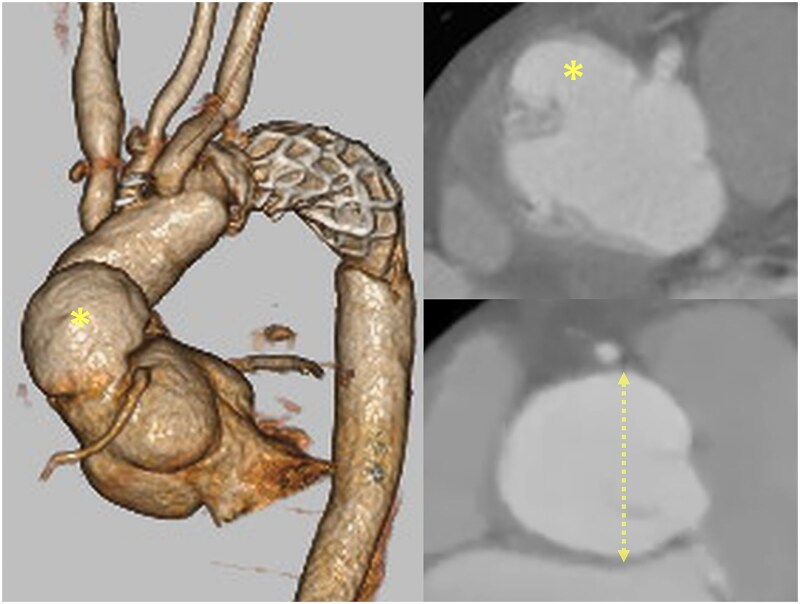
Preoperative CT showing the pseudoaneurysm (asterisk) and aortic root dilation (dotted line; 46 mm).
